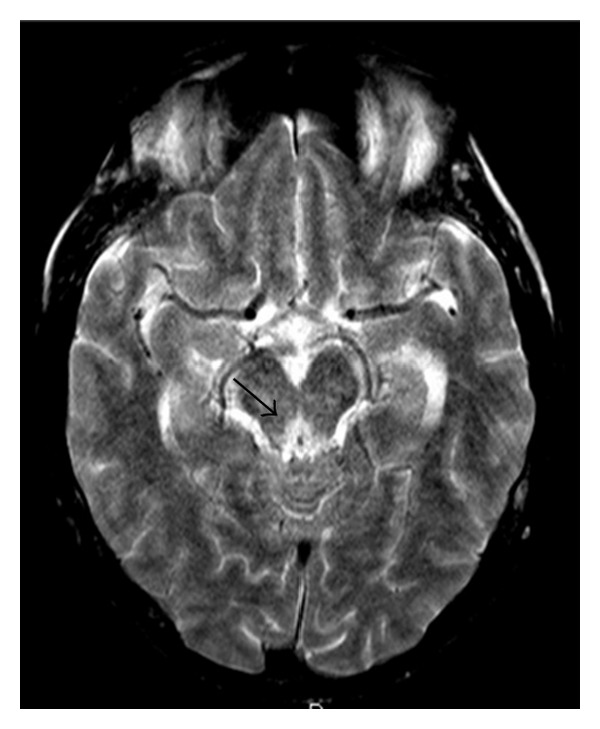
Sixty-four-year-old man with a 15-year history of alcohol abuse. T2-weighted axial image showing hyperintense signal alteration at the level of periaqueductal gray matter.
